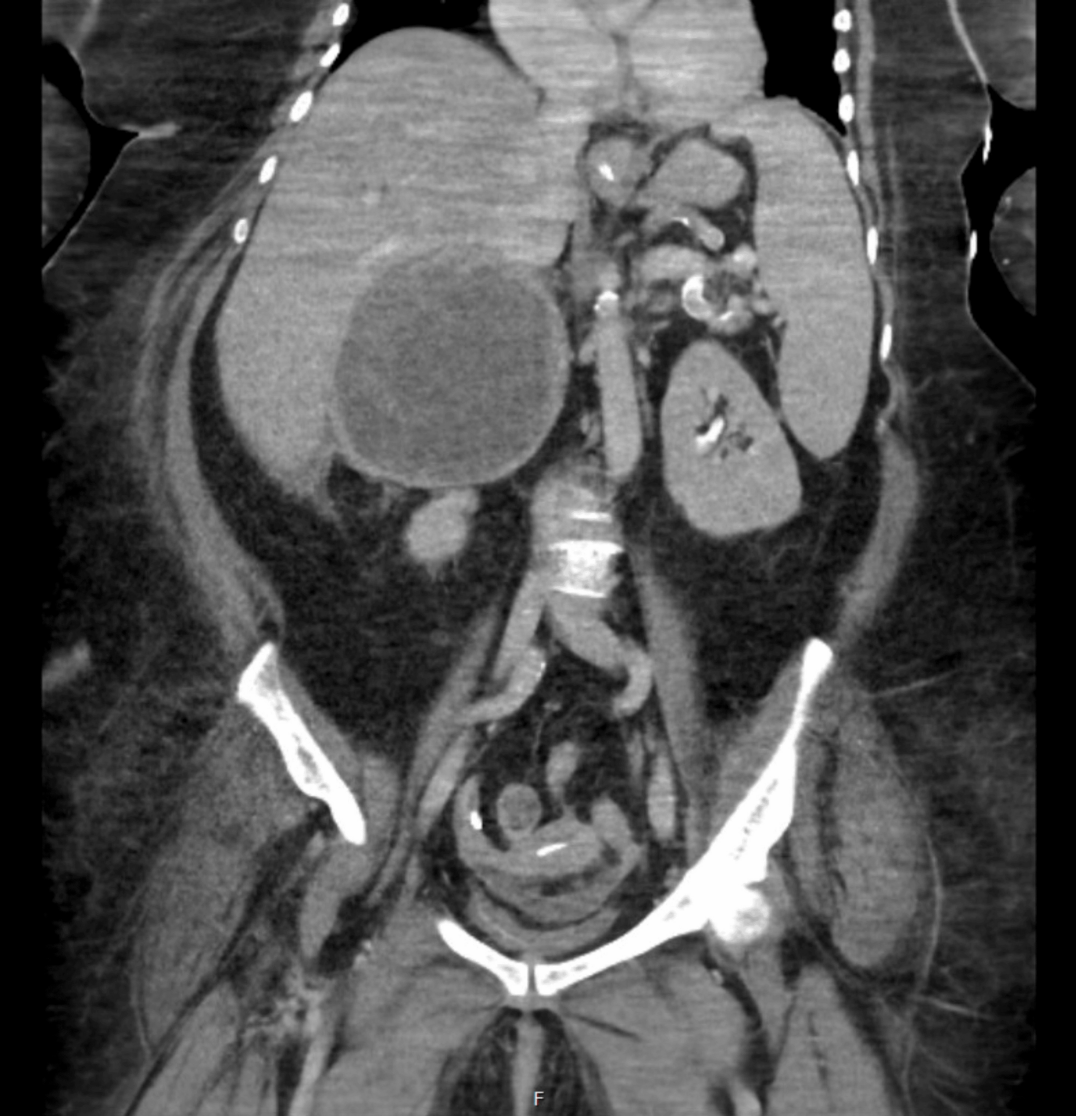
Representative coronal slide of abdominal computed tomography revealing a large right-sided adrenal abscess
